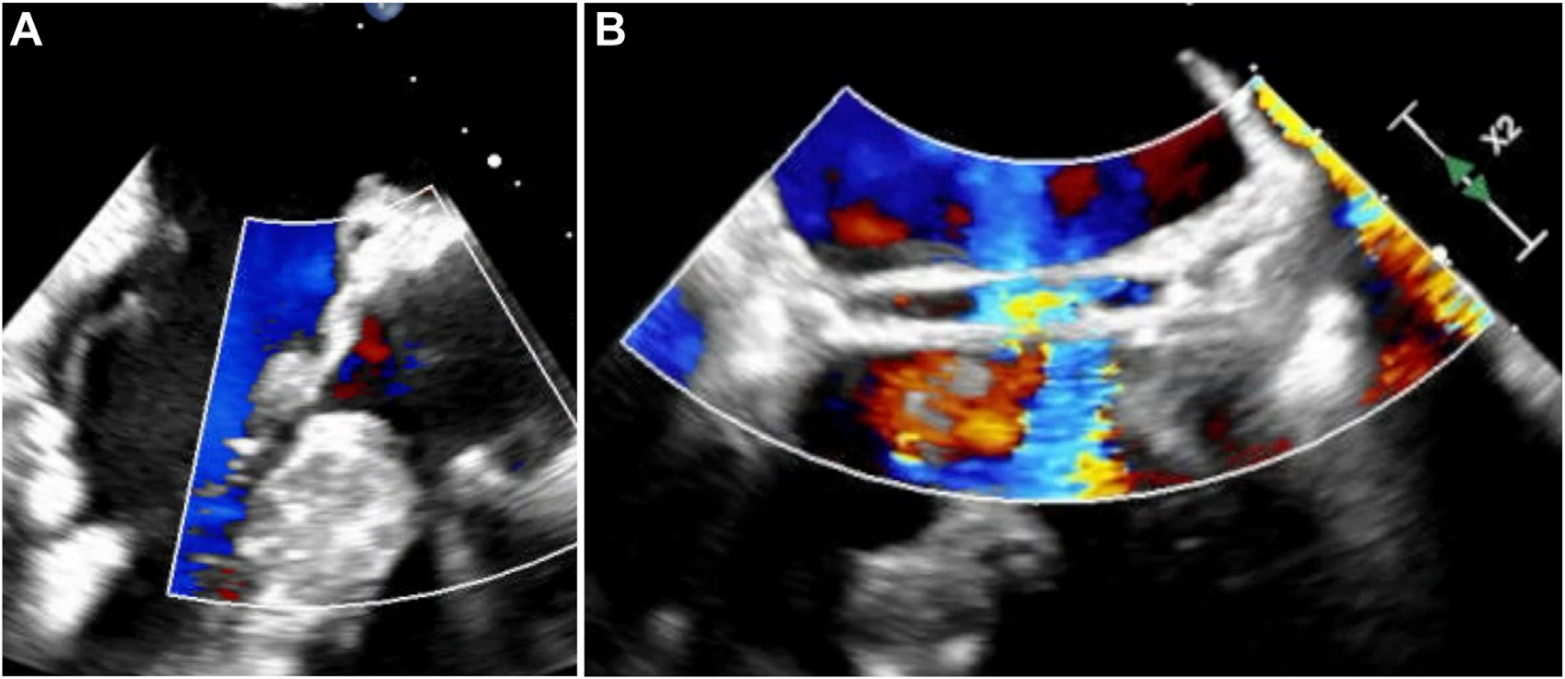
Postrepair transesophageal echocardiography (A) demonstrates preservation of the anomalous left circumflex, with the graft well-seated down to the annular plane, and (B) confirms flow in the left circumflex after valve-sparing root replacement.
